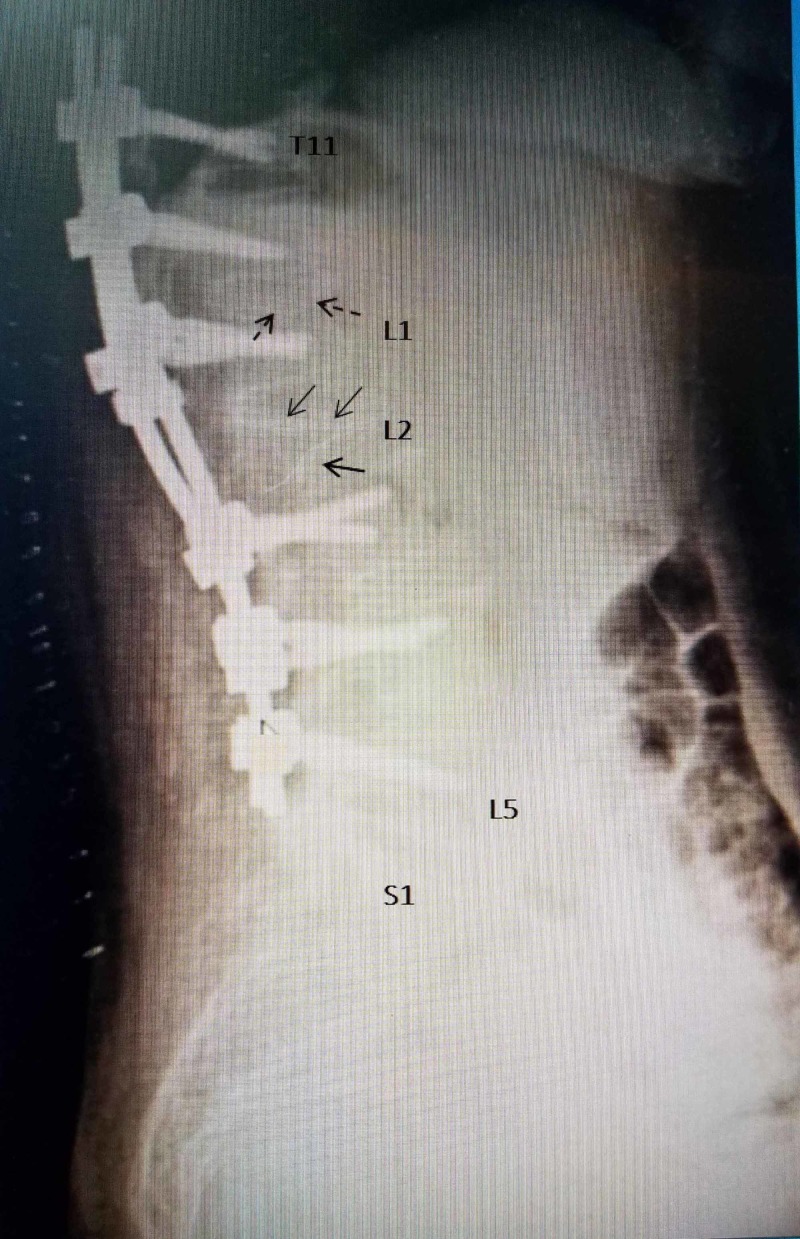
Postoperative lateral X-ray showing screw placement Postoperative lateral radiograph showing the pedicle screws and rods from T11 to L5. The collapsed L2 vertebrae (solid black arrows) is bridged by the screws three segments above and below the fracture and the preoperative kyphosis is reduced closer to normal. The superior endplate of L1 is also partially collapsed (dashed black arrow).
